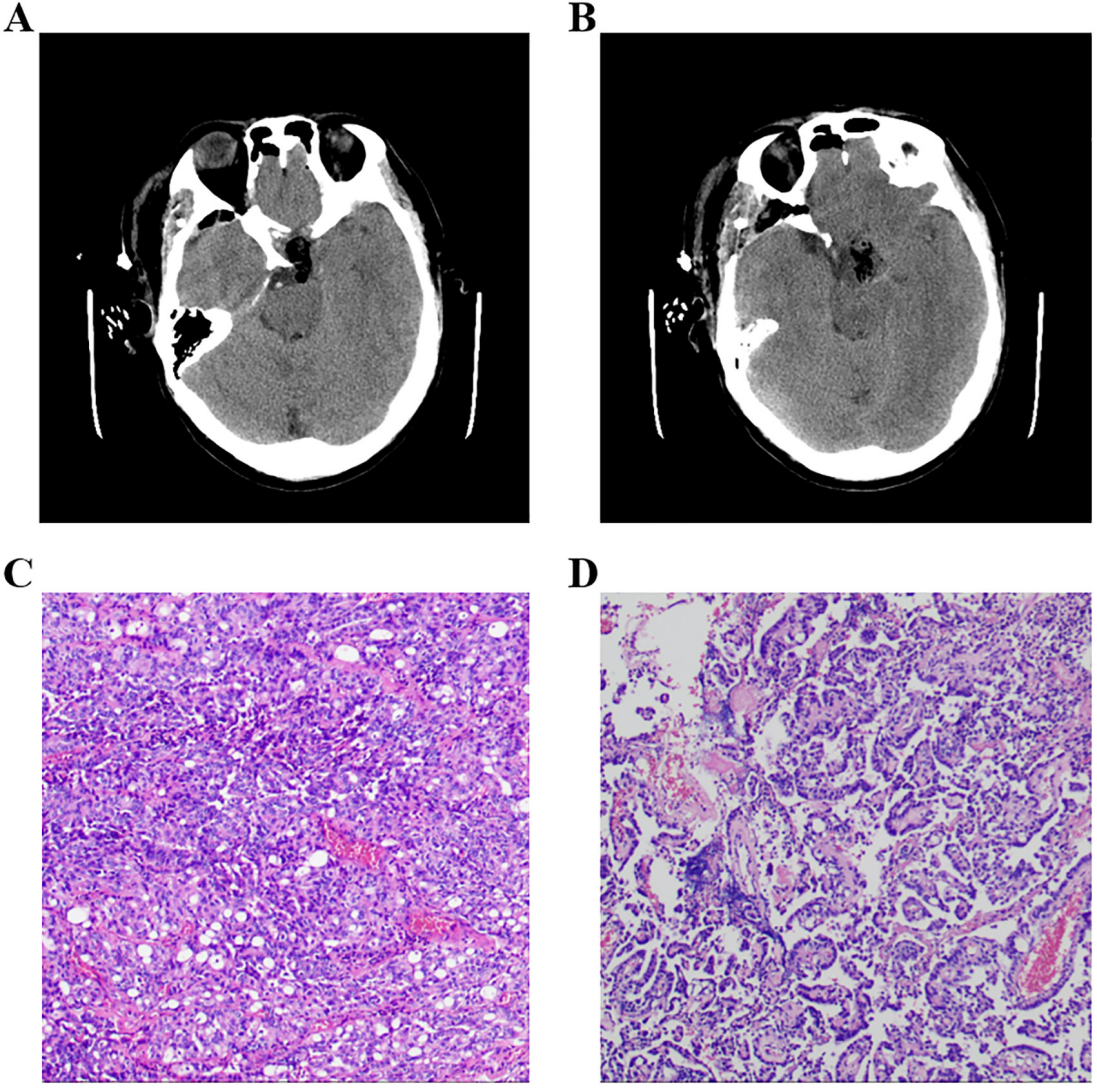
(A, B) Postoperational CT examination of the head. (C, D) Pathology results of the tumor, and the immune markers were as follows: ACTH(−), CD56(+), CK(+), CK20(−), CK8/18(+), CgA(−), FSH(−), GFAP(−), GH(−), Ki-67(+,3-5%), LH(−), NSE(+), P53(+), P63(−), PIT-1(−), PR(−), PRL(−), S-100(+), SF-1(−), Syn(−), TSH(−), TTF-1(−), Tg(−), Villin(−), Vim(+), and WT-1(+). ACTH, adrenocorticotropic hormone; PRL, prolactin; GH, growth hormone; TSH, thyroid-stimulating hormone; FSH, follicle-stimulating hormone; LH, luteinizing hormone.
